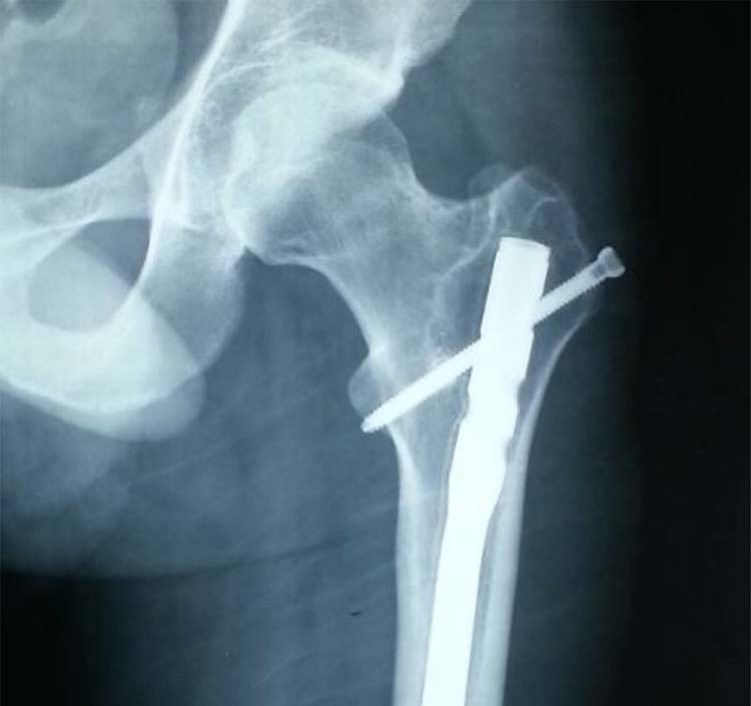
Anteroposterior radiograph showing a buried intramedullary nail.
